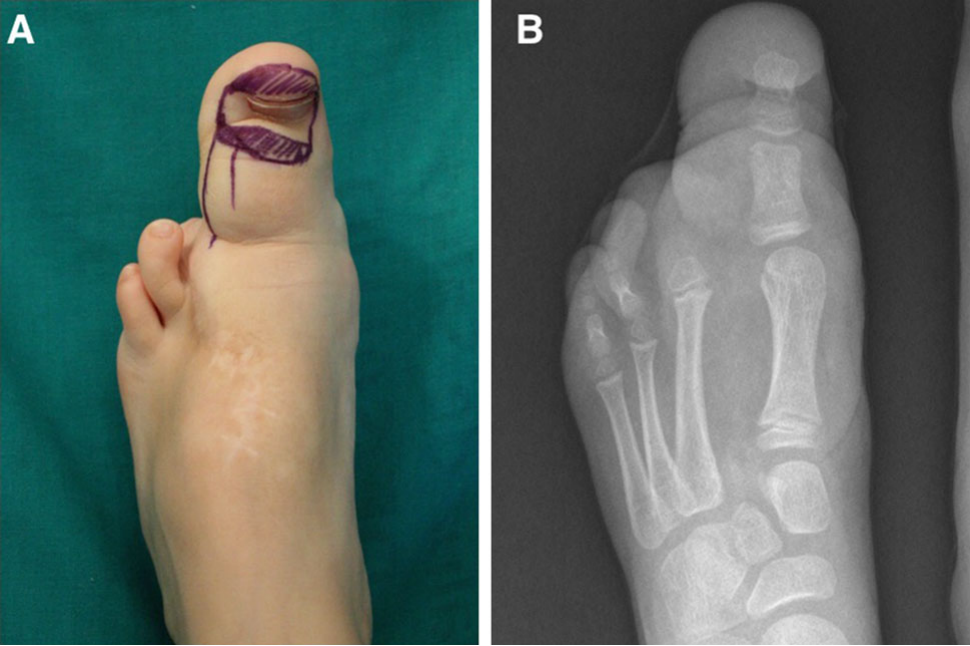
Case 2: Initial clinical photograph (a) and radiograph (b)
